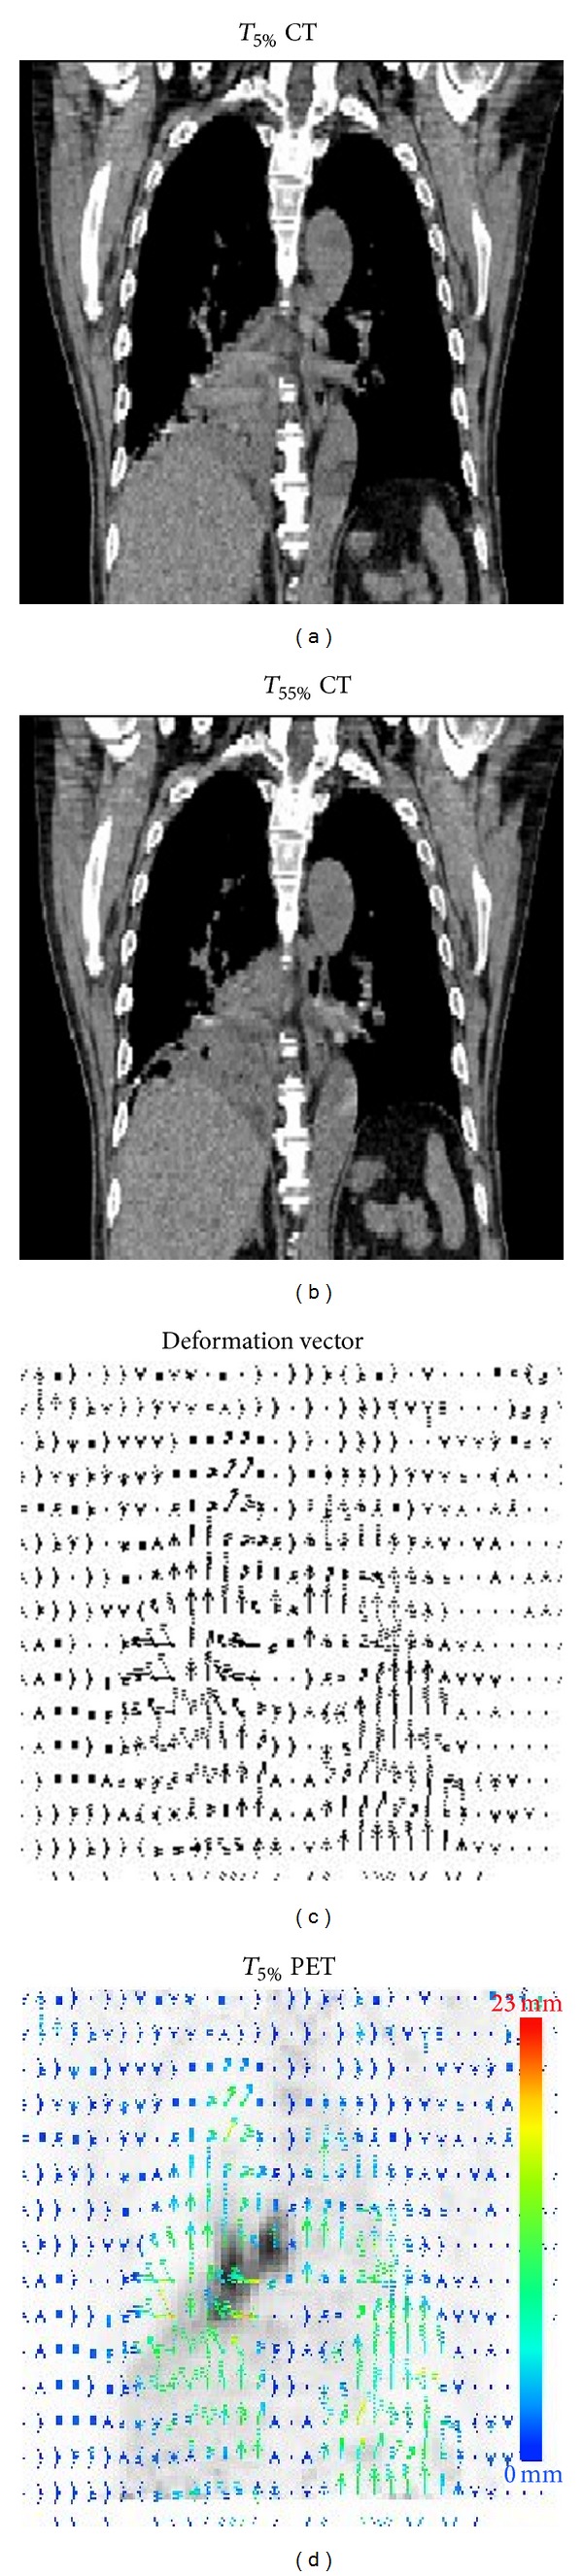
Example of deformable image registration using the 3D optical flow method to map the T5% PET to T55% PET. (a) A coronal view of the normal end-inspiration CT (T5%, original image) of 4D-CT. (b) The corresponding slice of the location in (a) at end-expiration CT (T55%, reference image) of 4D CT. (c) The calculated deformation vectors for the registration of original image to the reference. (d) The corresponding T5% PET with the 3D OFM deformation vectors superimposed.
